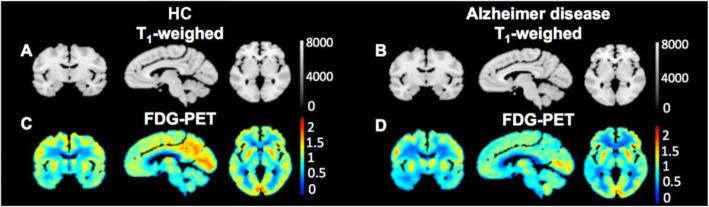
Modality of PET/CT. The standardized glucose uptake value ratios (SUVR) relative to the cerebellum from 18F-FDG PET scans of a healthy control (HC) and an Alzheimer’s disease patient. Adapted with permission from *Kazemifar et al.*
